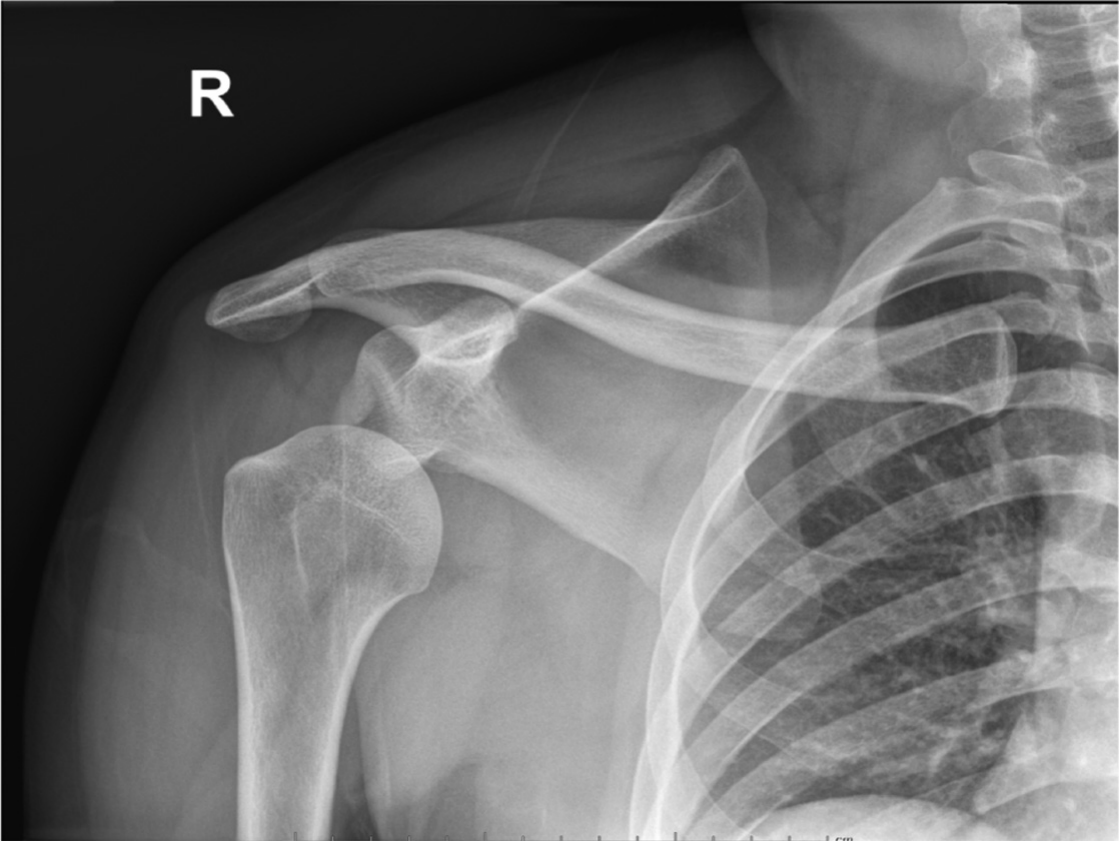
Radiograph of anterior shoulder dislocation, courtesy of Nirav Joshi, MD, Mount Sinai Medical Center, Miami Beach.
